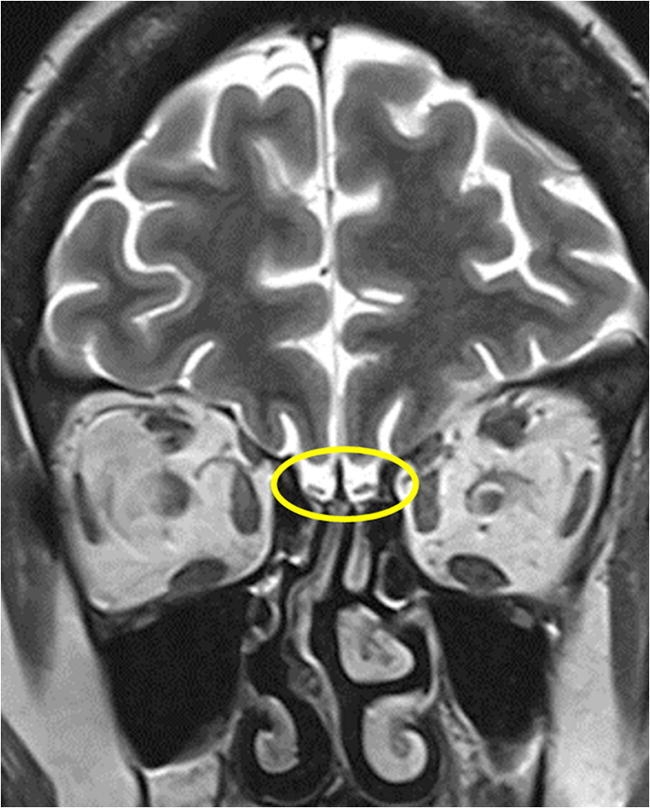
Coronal T2-WI MR image in a patient after COVID-19 and hyposmia demonstrates bilateral atrophy of the olfactory bulb (yellow circle)
